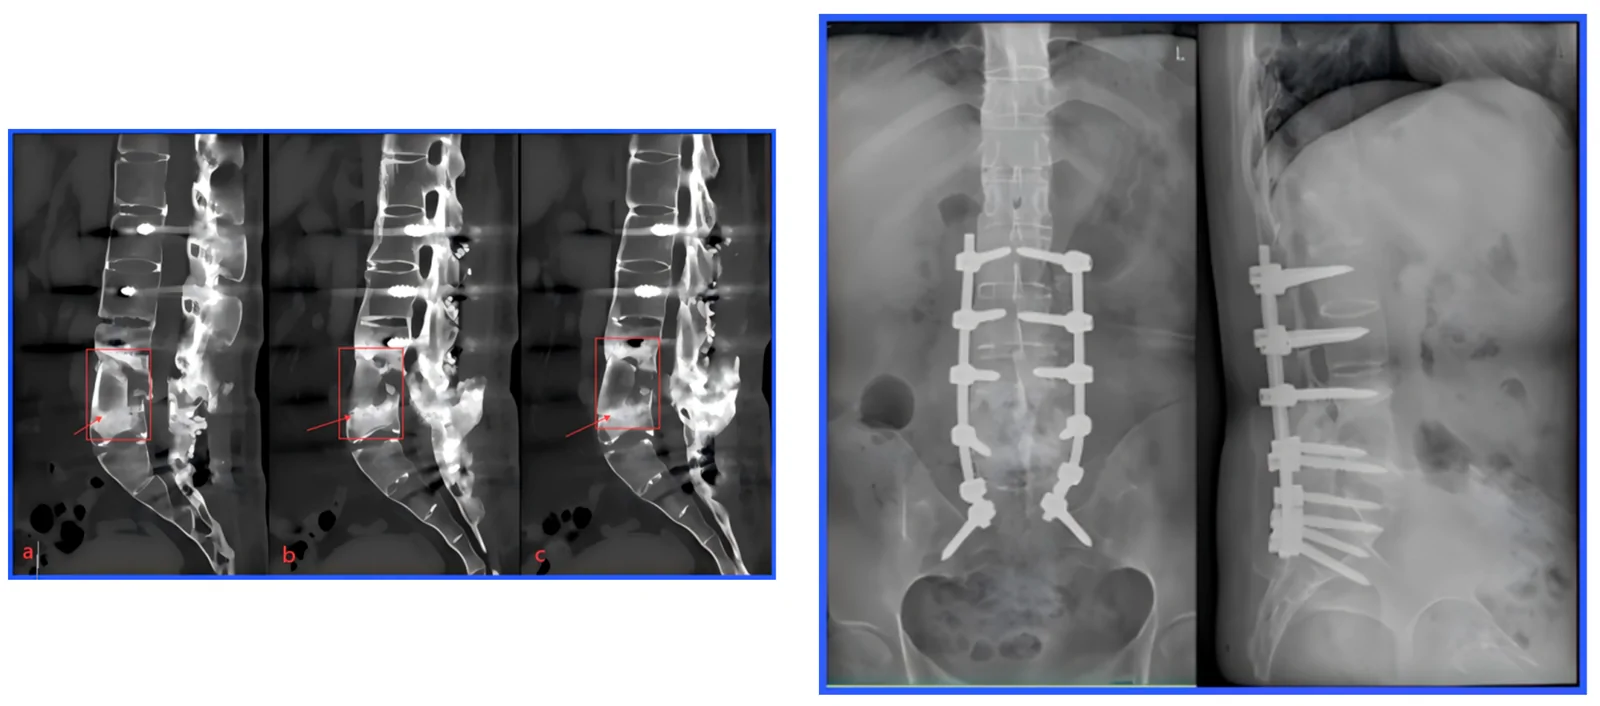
Imaging at the 3-month follow-up. (a) Anteroposterior radiograph shows maintained alignment and stable instrumentation without evident implant failure. (b) Lateral radiograph shows maintained alignment and stable instrumentation without evident implant failure. (c) Computed tomography demonstrates progressive trabecular–osseous bridging across the grafted L4/5 segment, consistent with early fusion progression rather than definitive mature fusion. Red boxes indicate the grafted L4/5 anterior column region, and red arrows indicate progressive trabecular–osseous bridging between the structural graft and the adjacent vertebral bodies.
